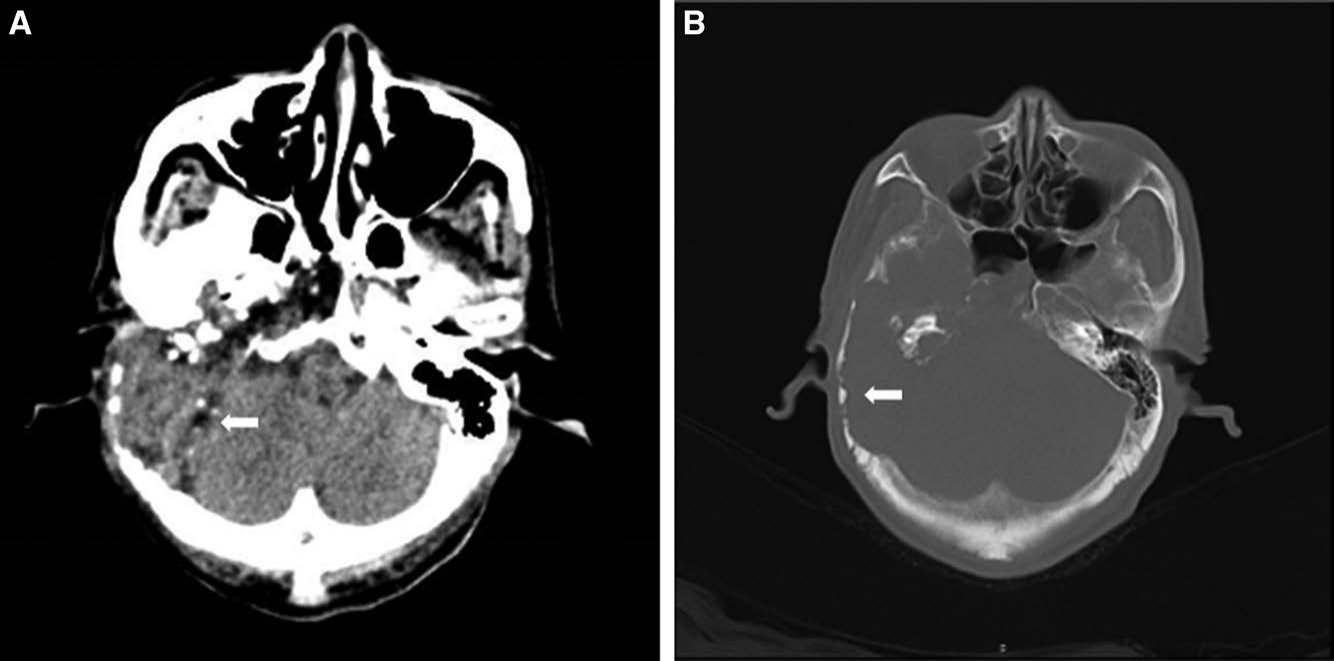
Preoperative computed tomography (A and B) revealed bone destruction of the right temporal bone, which was filled with soft tissue density, encroaching on the right inner and middle ear structures, encompassing the right internal carotid artery, partially entering the sphenoid sinus, and destroying the occipital bone posteriorly.
